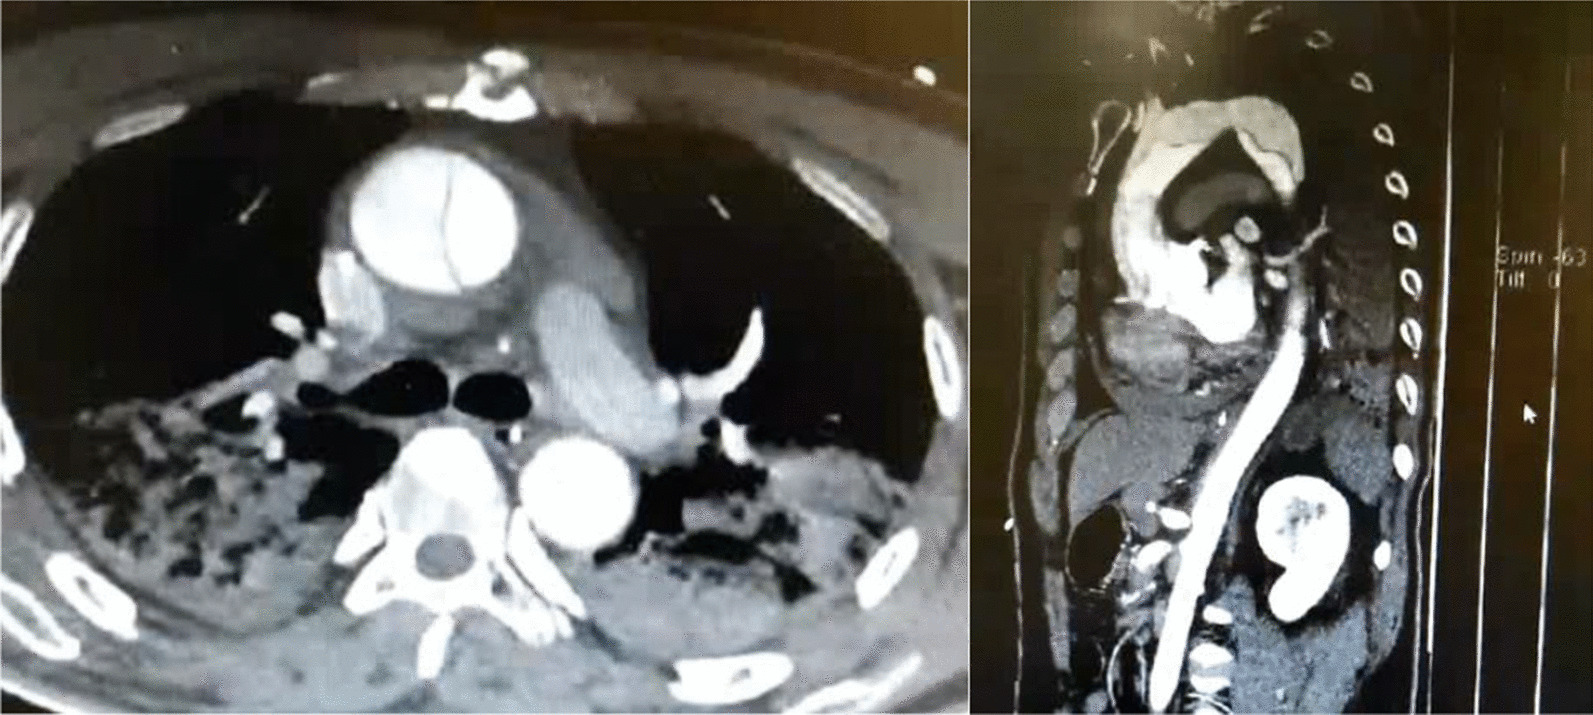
Computed tomography scan of the chest
